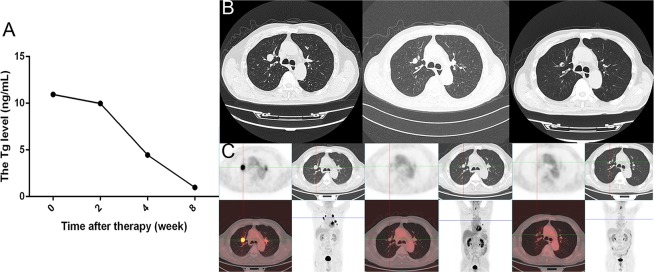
The Tg, CT, and PET/CT results obtained before and after apatinib therapy in a male patient A. Tg levels at baseline and after therapy. B. CT images at baseline and after 4 and 8 weeks of apatinib therapy. C. PET/CT images at baseline and after 4 and 8 weeks of apatinib therapy. Tg, thyroglobulin; CT, computed tomography; PET/CT, positron emission tomography/ computed tomography.
